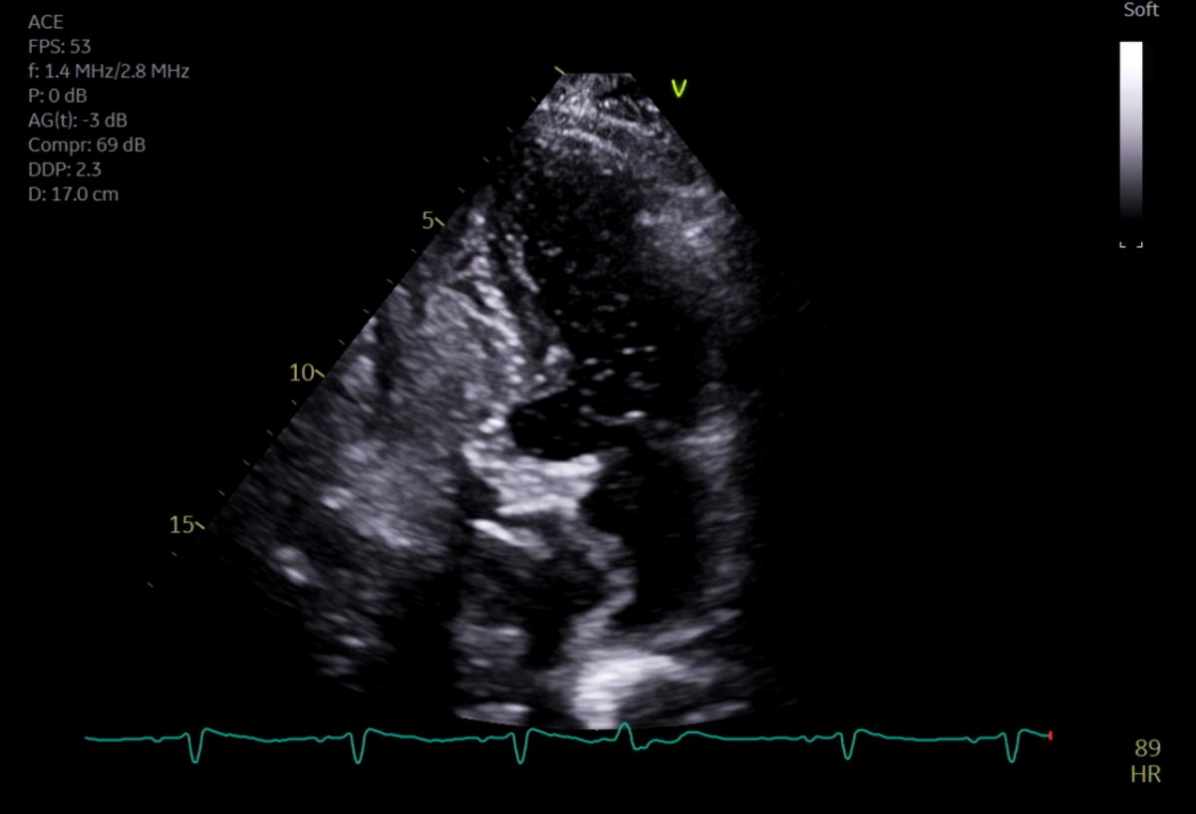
Transthoracic apical four-chamber image obtained in the upright position. The atrial septum is aneurysmal and bows towards the left atrium, suggesting an interatrial pressure gradient. Intravenous agitated saline has been administered and opacifies the right atrium and ventricle. Saline microbubbles are noted in the left ventricle, consistent with an intracardiac right to left shunt.
